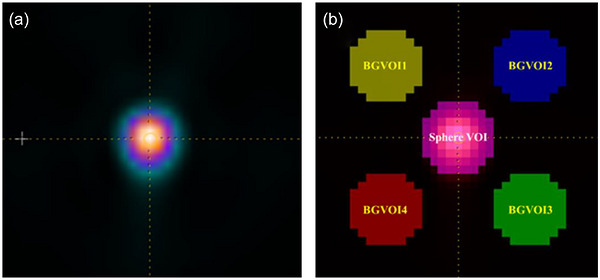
(a) Transaxial slice of Tb‐161 sphere SPECT image, (b) VOIs on that image.
